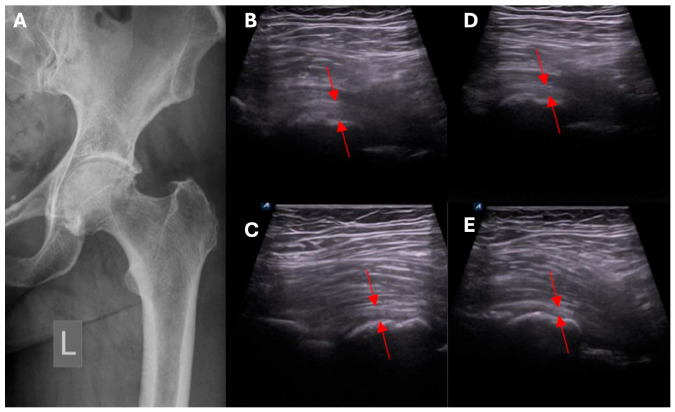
(A) Radiological image of the hip joint. (B–E) Ultrasound examination of the hip joint; red arrows indicate gradual narrowing of the front joint capsule over time following the administration of NSBT. L—left side.
